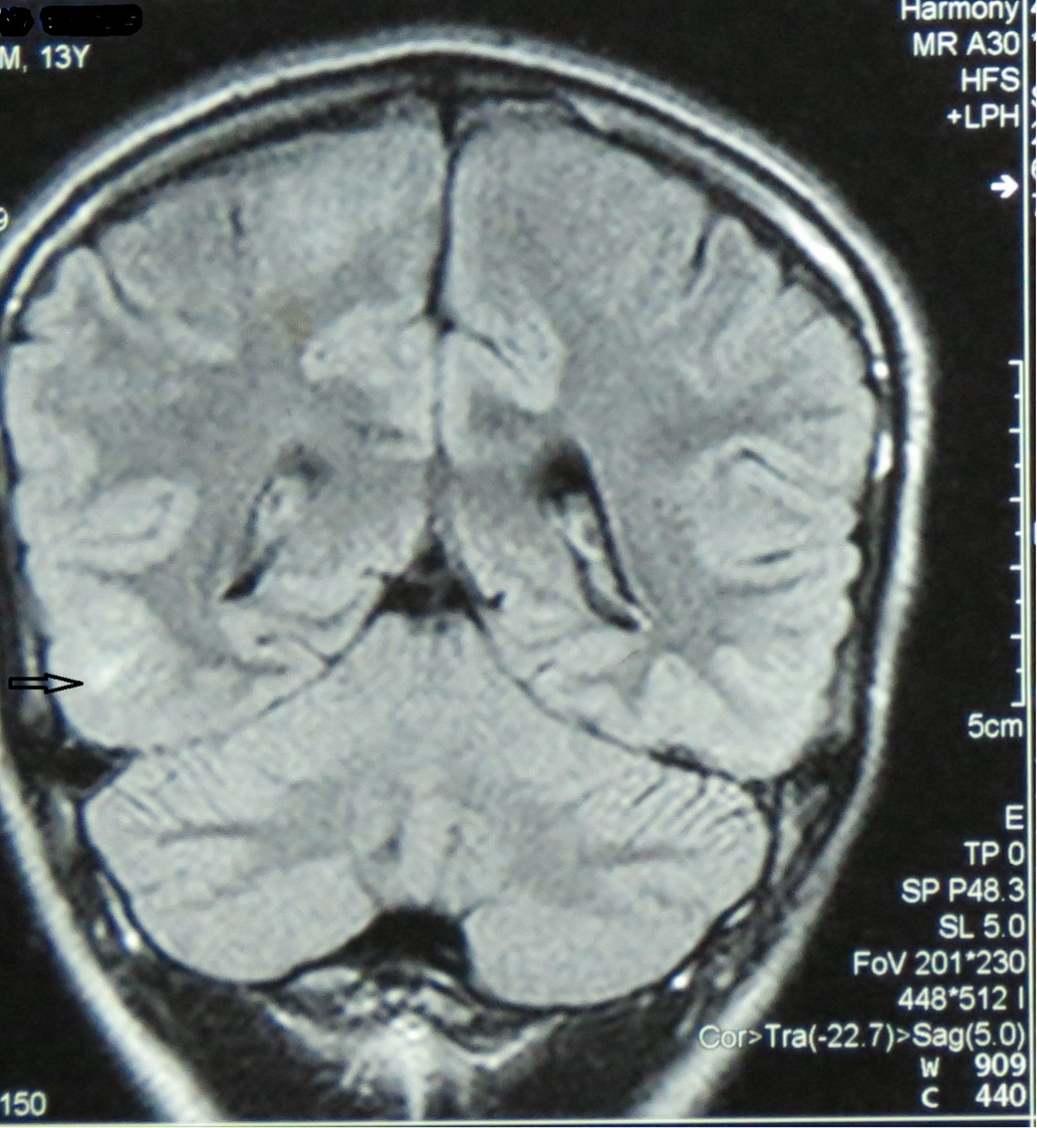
Magnetic resonance imaging scan done day-10 of the admission showed linier sub-cortical hyper intensities (arrow) in both parietal regions.
